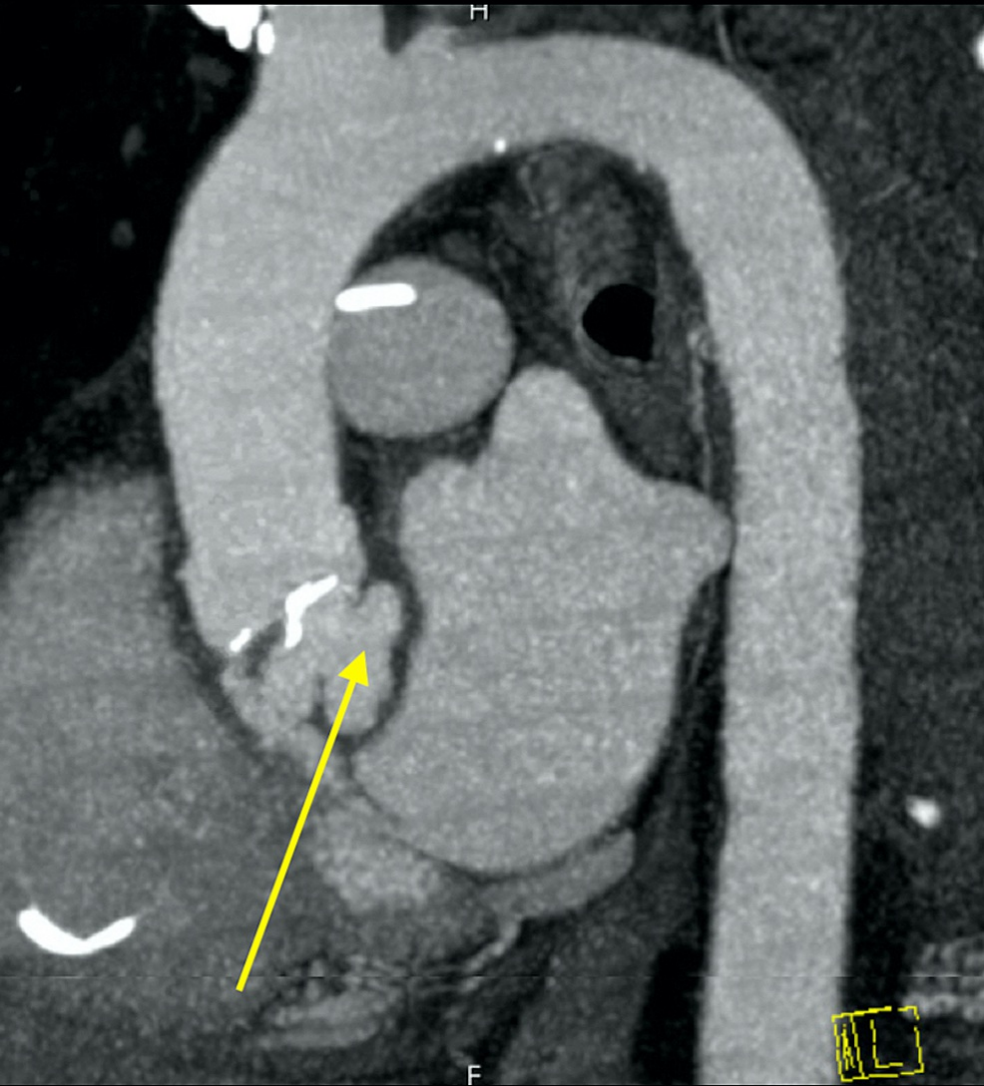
Gated coronary computed tomography with further visualization of aortic root abscess (yellow arrow).
